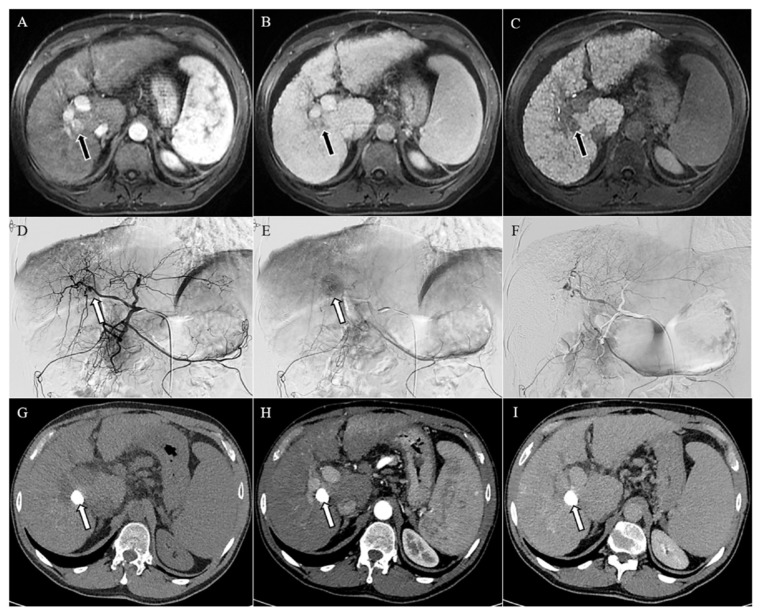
Magnetic resonance imaging (A–C) showing a lesion of 18 mm in the liver segment 8, hypervascularized in the arterial phase (arrow in (A)), with washout of the contrast media in the venous phase (arrow in (B)), and hypointense in the hepatobiliary phase (arrow in (C)), consistent with hepatocellular carcinoma in the context of cirrhosis. Angiographic study (D–F) of the liver confirmed the hypervascular lesion in the early and late arterial phases (arrows in (D,E), respectively), which was no longer visible after chemoembolization (F). Computed tomography (G–I) performed one month after chemoembolization demonstrated an homogeneous accumulation of the lipiodol in the unenhanced image (arrow in (G)), with no signs of relapse in the arterial and delayed phases (H,I).
